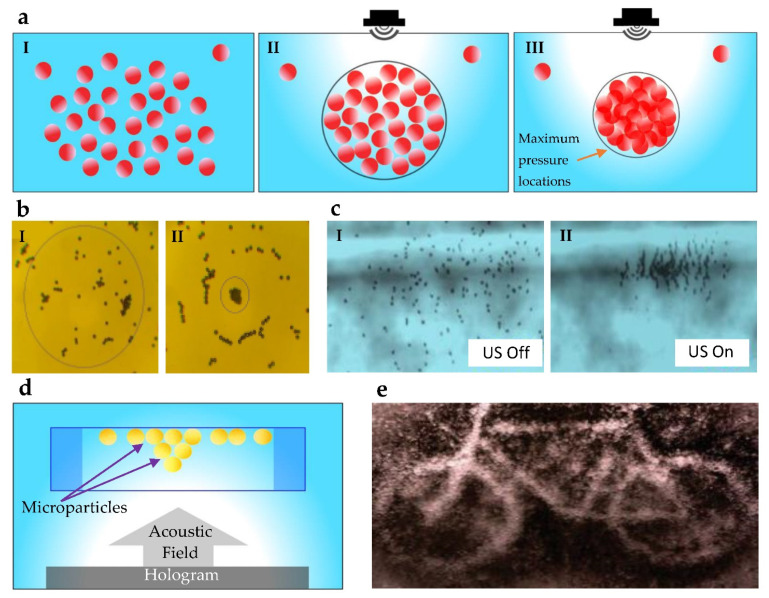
Shape formation techniques using acoustic fields: (a) Under an applied acoustic field, particles move towards minimum pressure locations. Circle diameter variation shows the convergence of pressure maxima on the water surface. (b) Acoustic traps generate the formation of microparticles by creating pressure minima surrounded by regions of a high pressure amplitude. Reproduced with permission from [69]; published by AIP Publishing. (c) Representative example of the rapid-ultrasound-triggered assembly of nanomotors into a swarm. Reproduced with permission from [48]; published by American Chemical Society. (d) An acoustic field is applied through the hologram to make target assemblies. (e) An arbitrary shape produced using travelling waves (TW) and holograms. Reproduced with permission from [75]; published by John Wiley and Sons.
